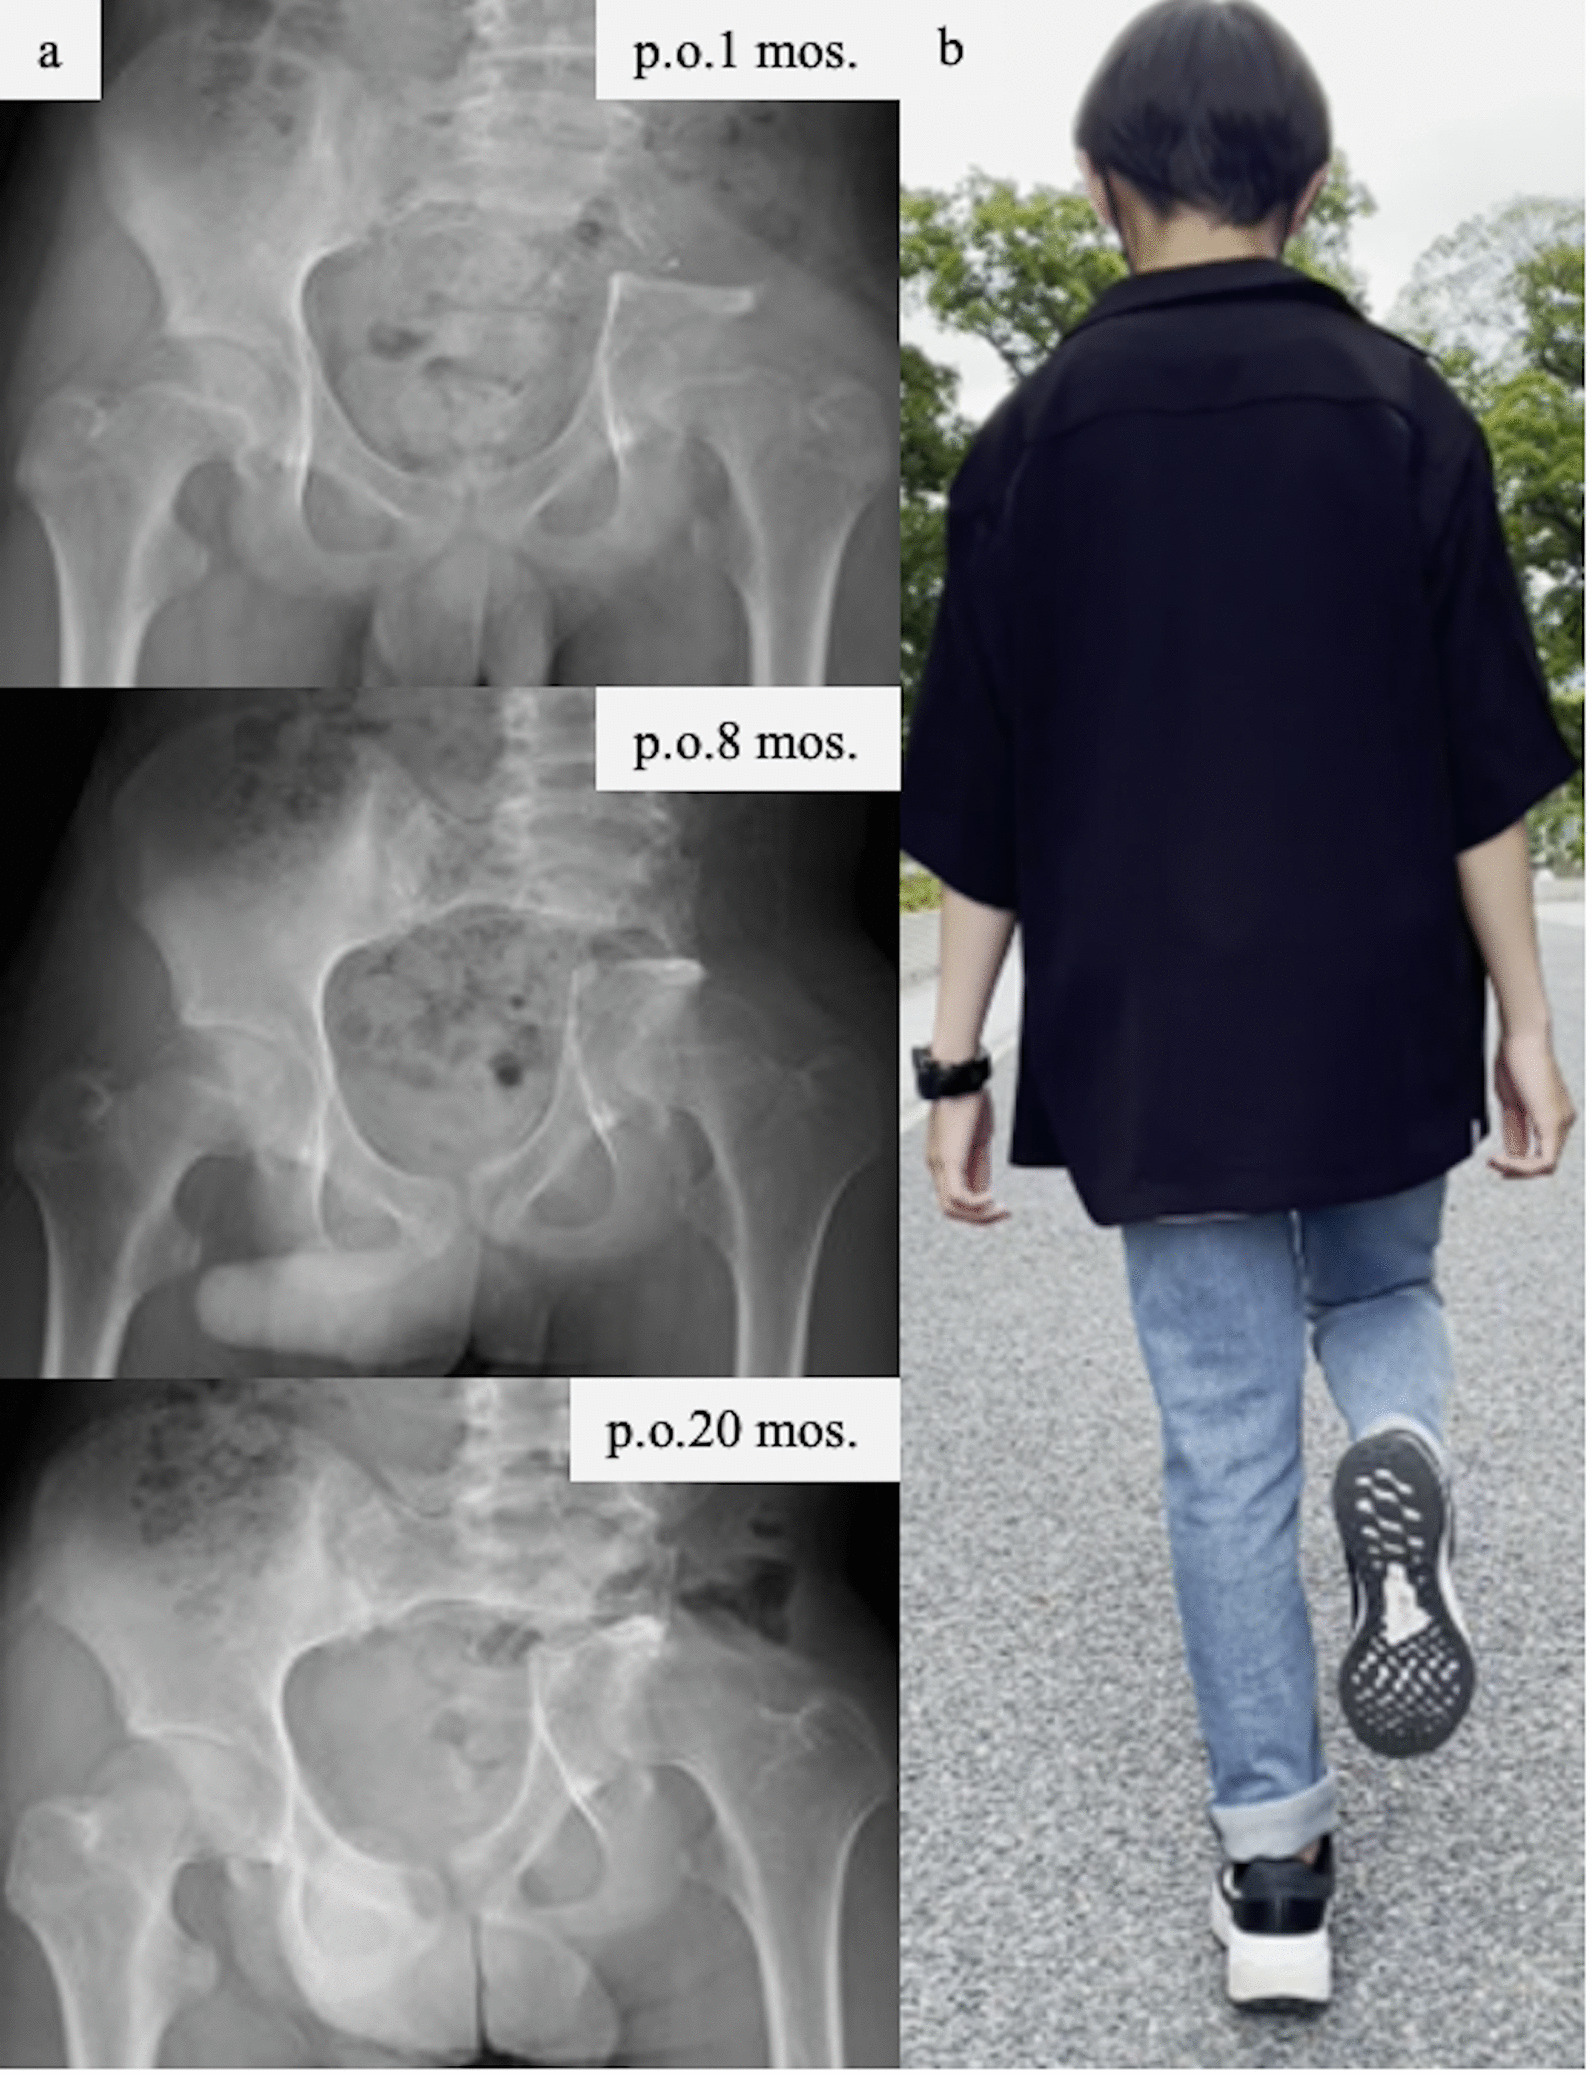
a Progress of postoperative plain X-ray imaging. The patient’s left hip was gradually centralized to the pelvic center. b There was no remarkable Trendelenburg sign at 20 months after the excision
